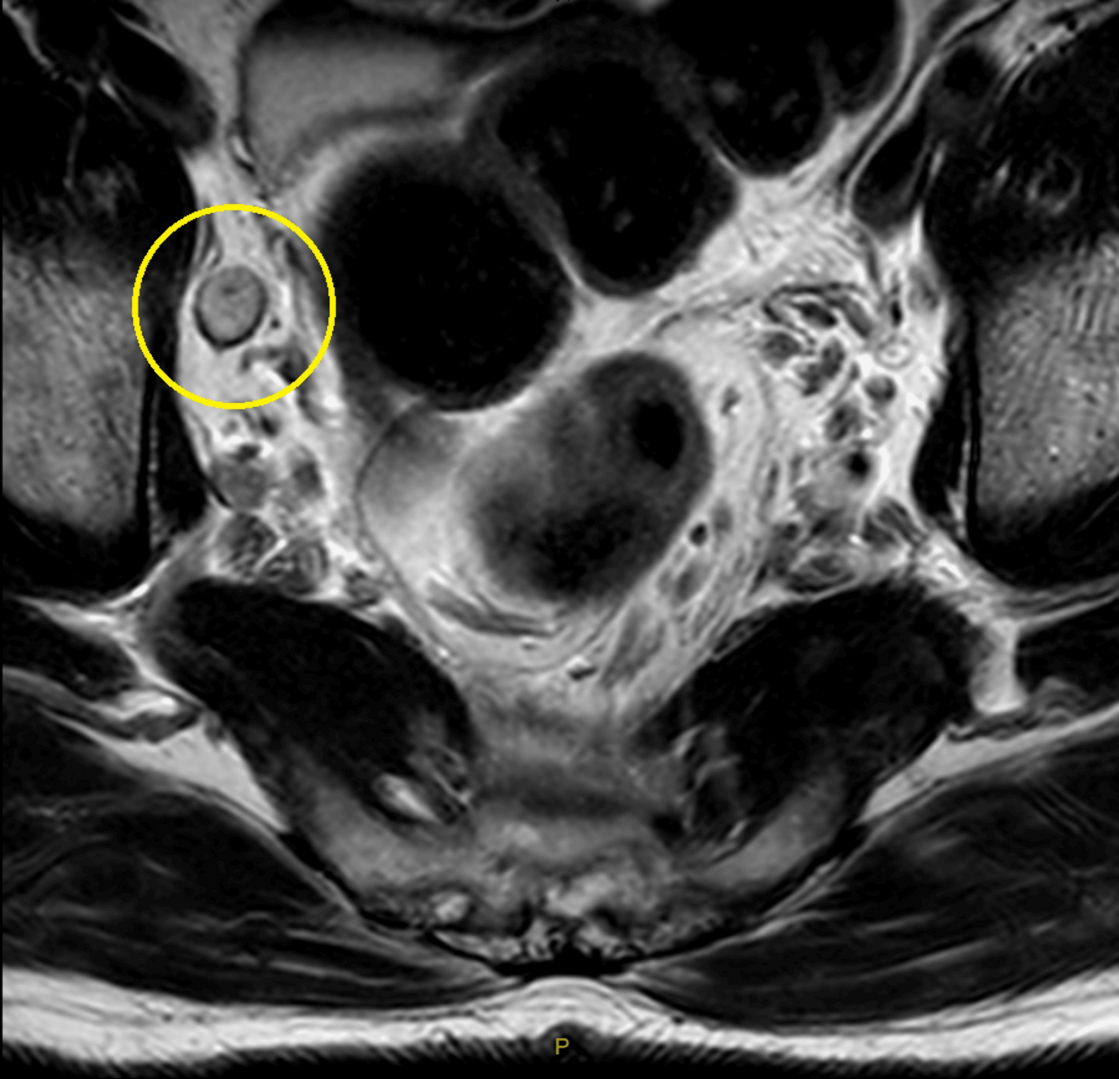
MRI scan of the pelvis with marked region highlighting a right pelvic side wall node.
